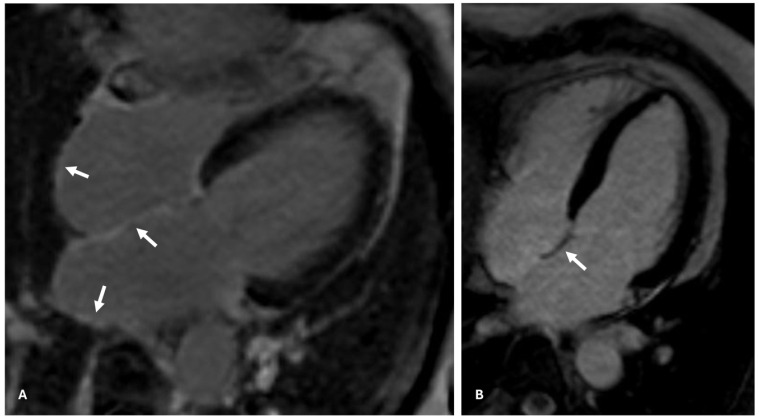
Delayed enhancement cardiac magnetic resonance imaging, long-axis 4-chamber view, in a patient with (arrows in (A)) and without (arrow in (B)) atrial fibrosis.
